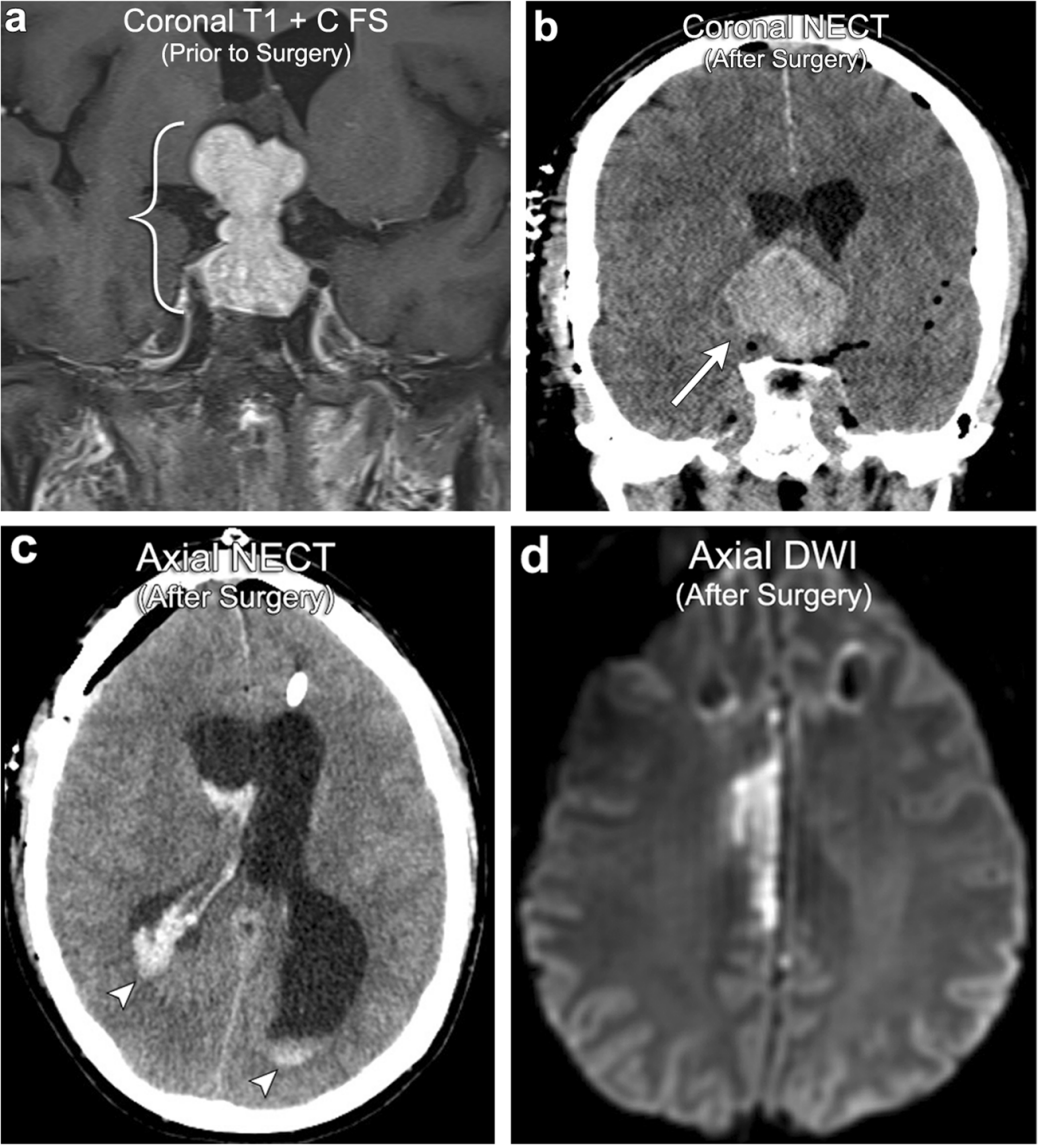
Postoperative complication: haemorrhage and infarct. A 52-year-old male with a large pituitary macroadenoma (bracket). Postoperative imaging after gross resection demonstrates haemorrhagic enlargement of the residual component of the adenoma consistent with apoplexy (arrow). There is also intraventricular extension of haemorrhage (arrowheads). Follow-up MRI demonstrates right anterior cerebral artery (ACA) territory infarction due to mass effect on the right ACA from the enlarged haemorrhagic pituitary adenoma
